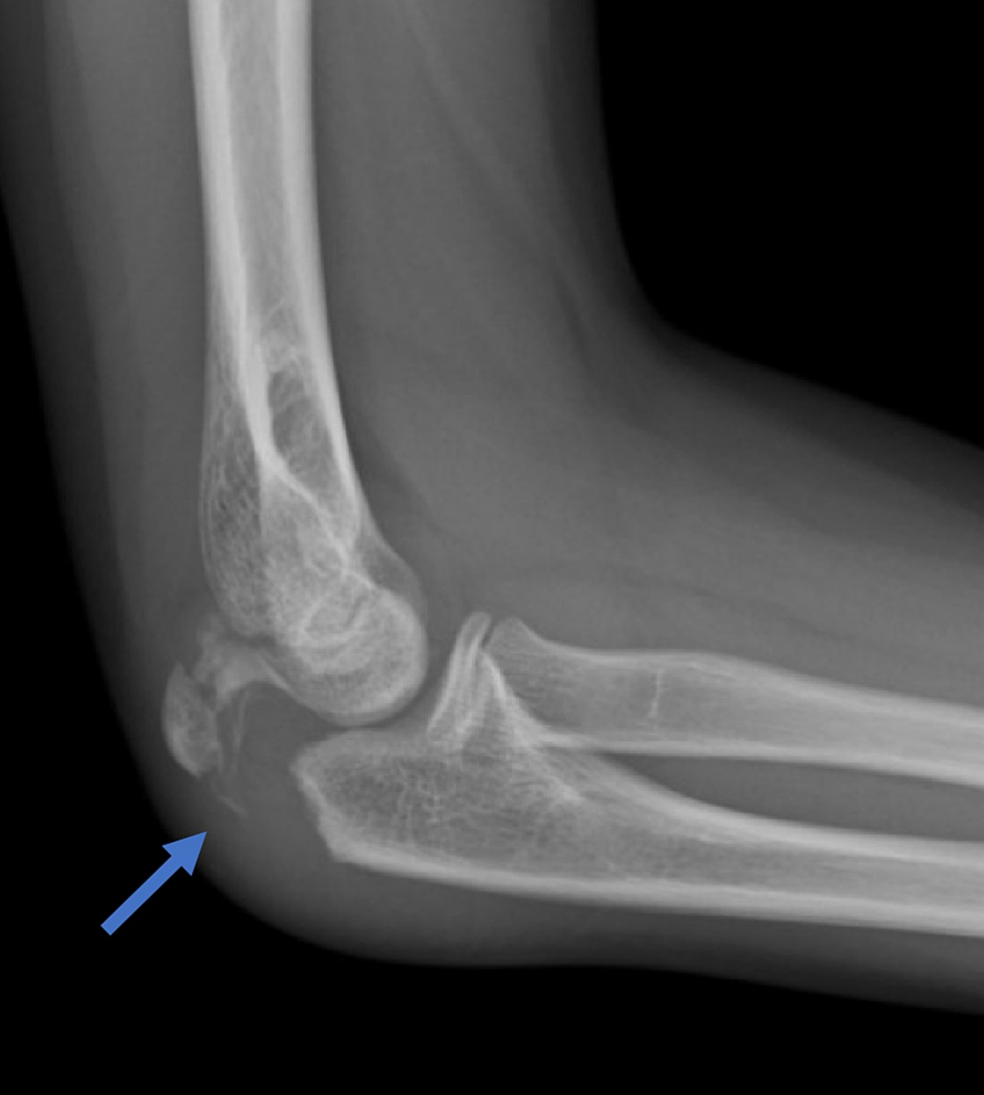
Lateral radiograph of the right elbow shows a displaced olecranon metaphyseal fracture (arrow).
